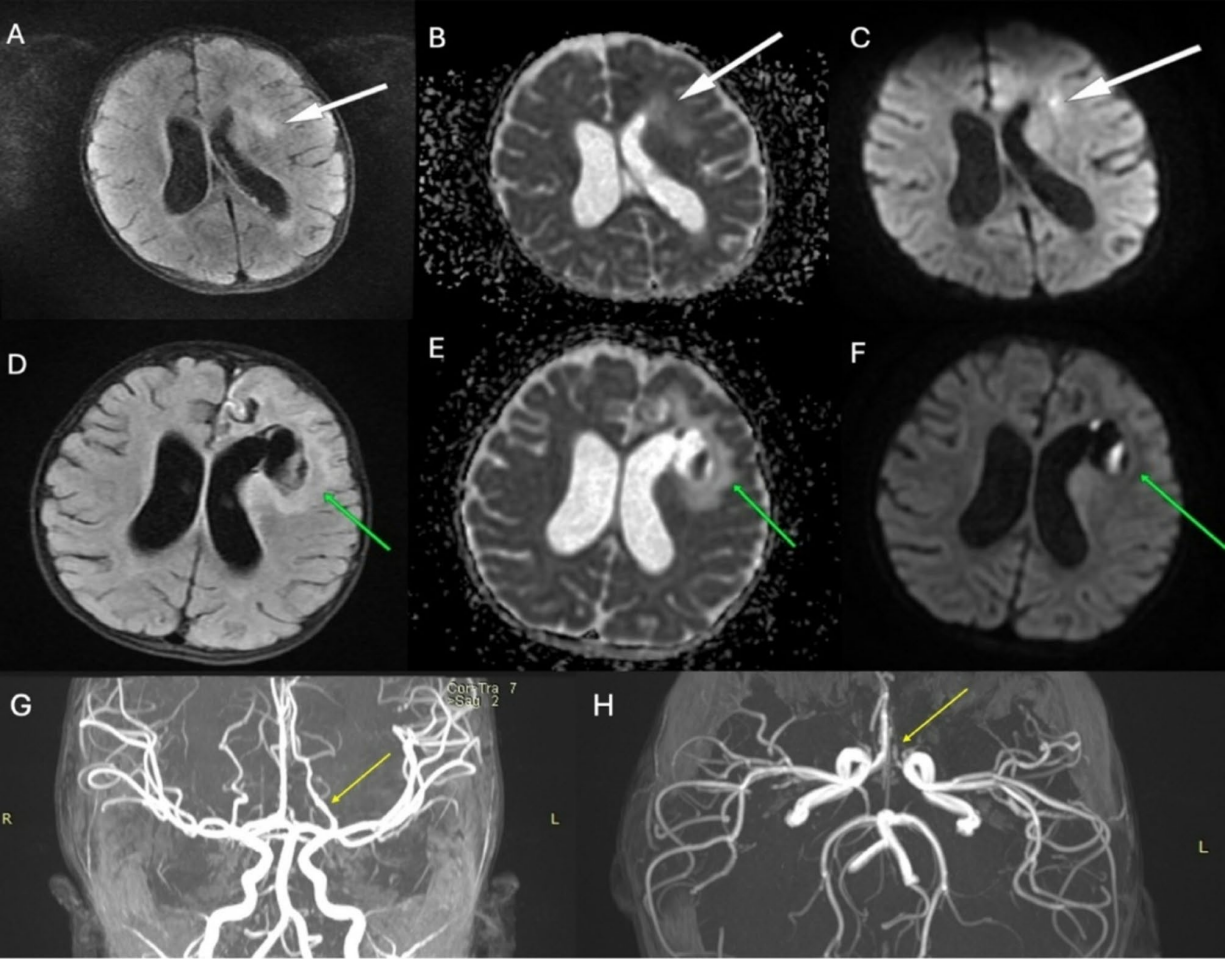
(A-B-C) Follow-up MRI showing hematoma resorption(White arrow) and no residual aneurysm filling (From left to right; T2, ADC, DWI). (D-E-F) Cerebral edema(Green arrow) near the hematoma was observed on the one-month follow-up MRI, though the patient’s neurological condition remained unchanged. (From left to right: T2, ADC, DWI). (G-H) MR angiography at 1-year follow-up revealed no residual aneurysm filling(yellow arrow)
